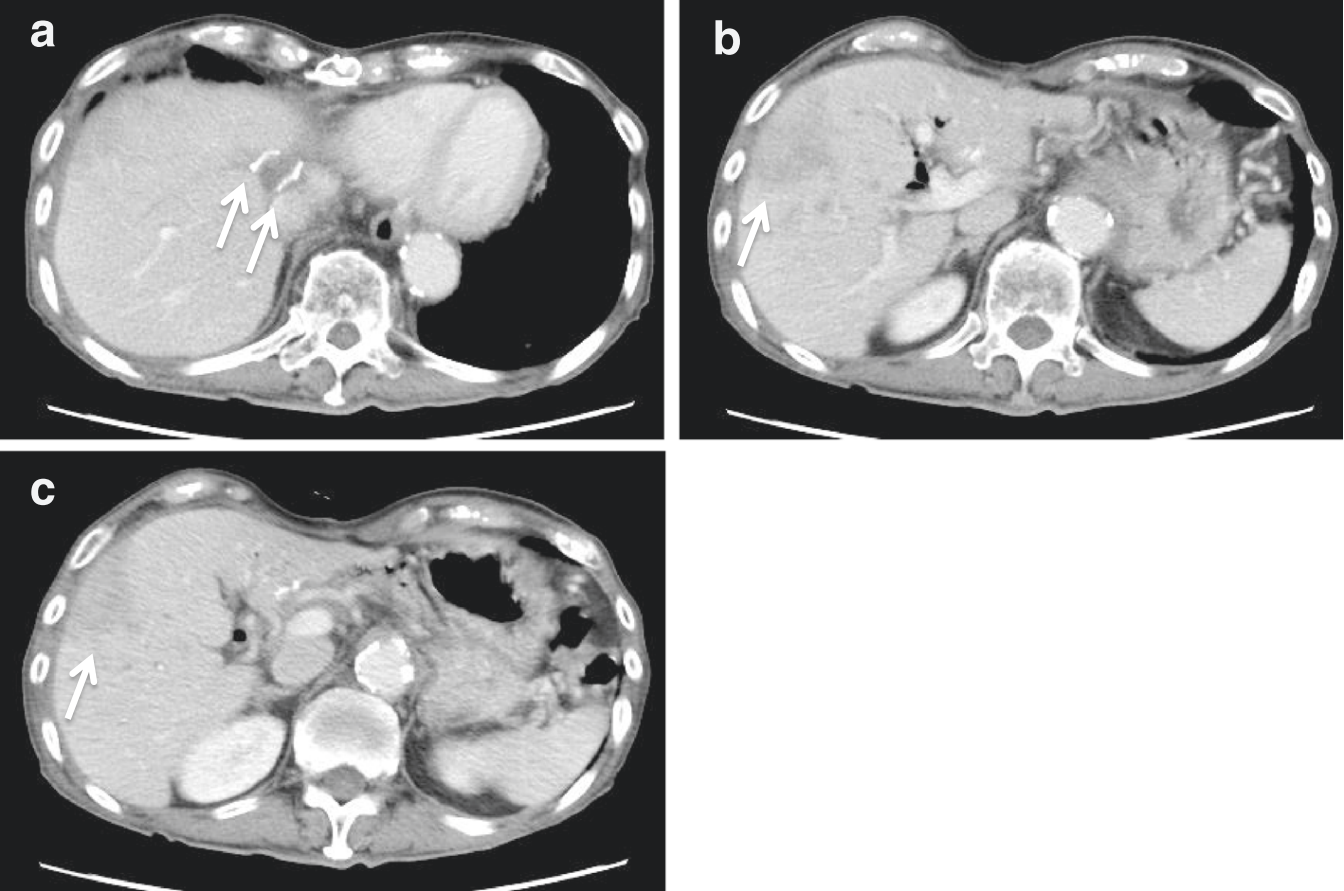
Follow-up computed tomography before discharge. a
